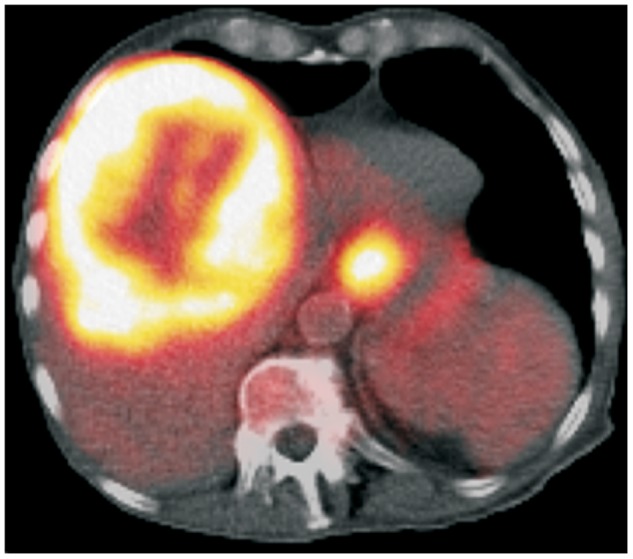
Positron emission/computed tomography scan reveals a large, intense metabolically active, low-density lesion with central photopenic area in the liver and an intense metabolically active area at the gastroesophageal junction.
